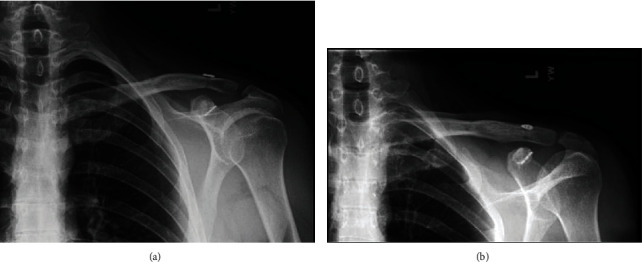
(a, b) Plain radiographs demonstrating well-aligned acromioclavicular and sternoclavicular joints without hardware complication.
